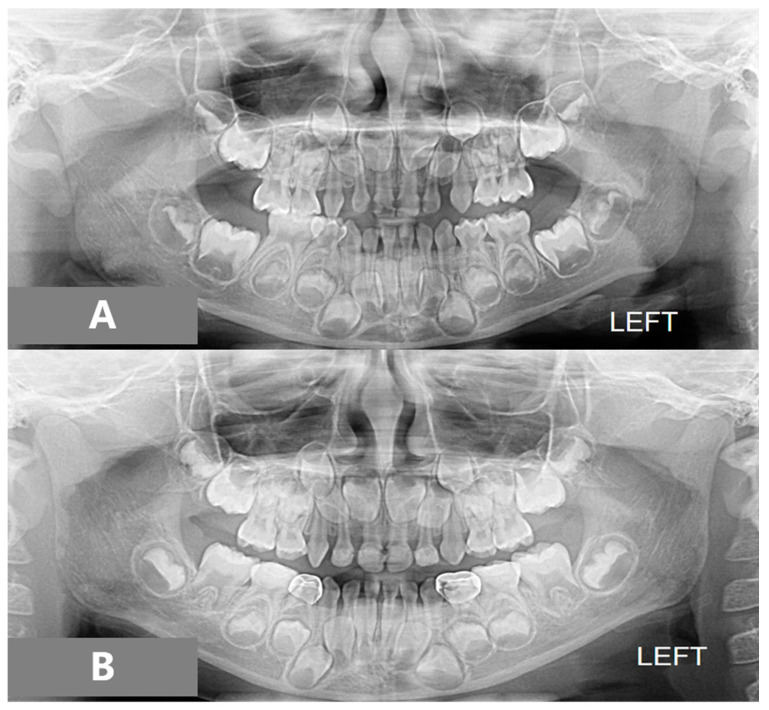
Panoramic radiographs: (A) pre-treatment, (B) post-treatment.
